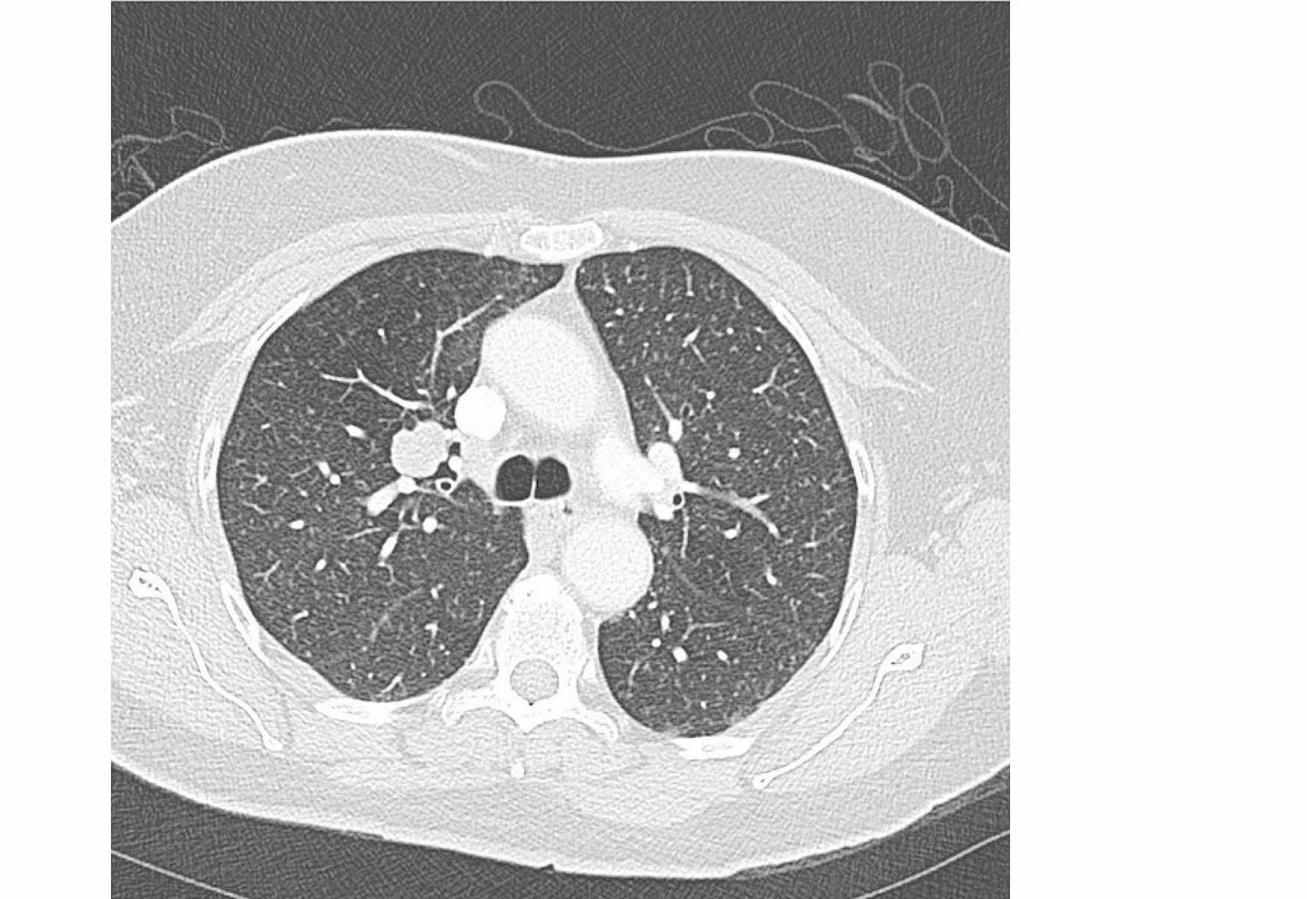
CT scan showing a mass with a dominant 2.1 × 1.7 cm solid component
